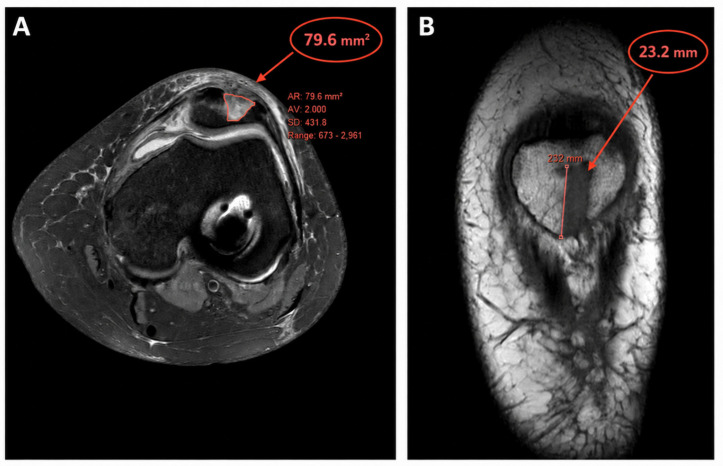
Patellar donor-site bone defect 4 weeks after anterior cruciate ligament reconstruction with a bone–patellar tendon–bone autograft: (A) axial AX PD SPAIR MRI showing the defect area used for base estimation; (B) coronal T1 TSE MRI showing the longitudinal extent of the defect.
